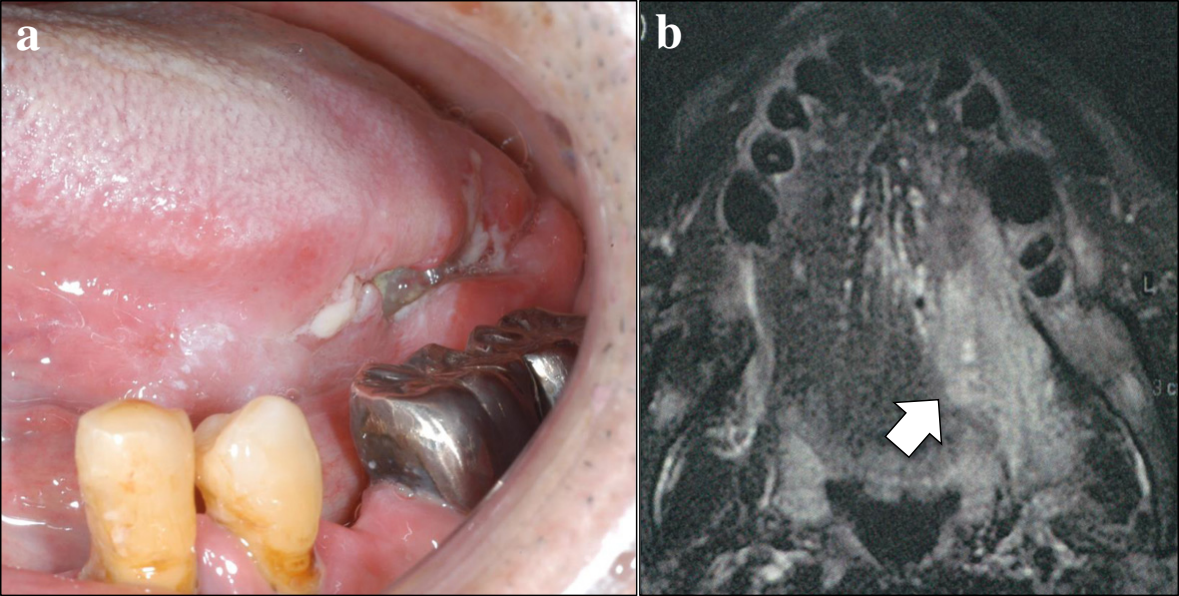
Intraoral and MRI findings. a Tumorous mass with ulcerative lesion on the left lateral border of the tongue. b Contrast-enhanced MRI findings. *White arrow* indicates tumorous mass
